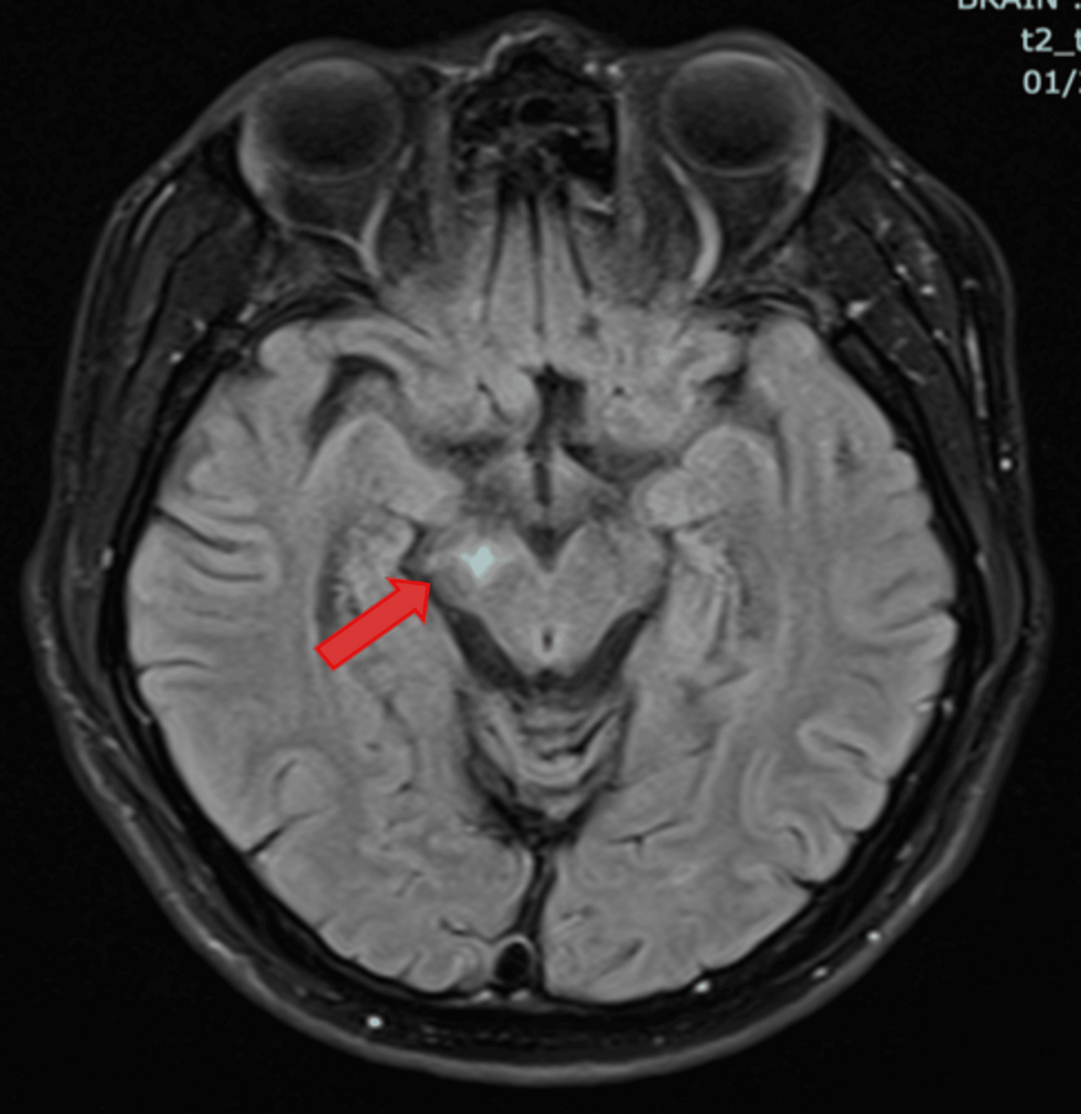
MRI of the brain with T2 FLAIR showed hyperintensity at the right cerebral peduncle (thick red arrow) T2-FLAIR: T2-weighted-Fluid-Attenuated Inversion Recovery
